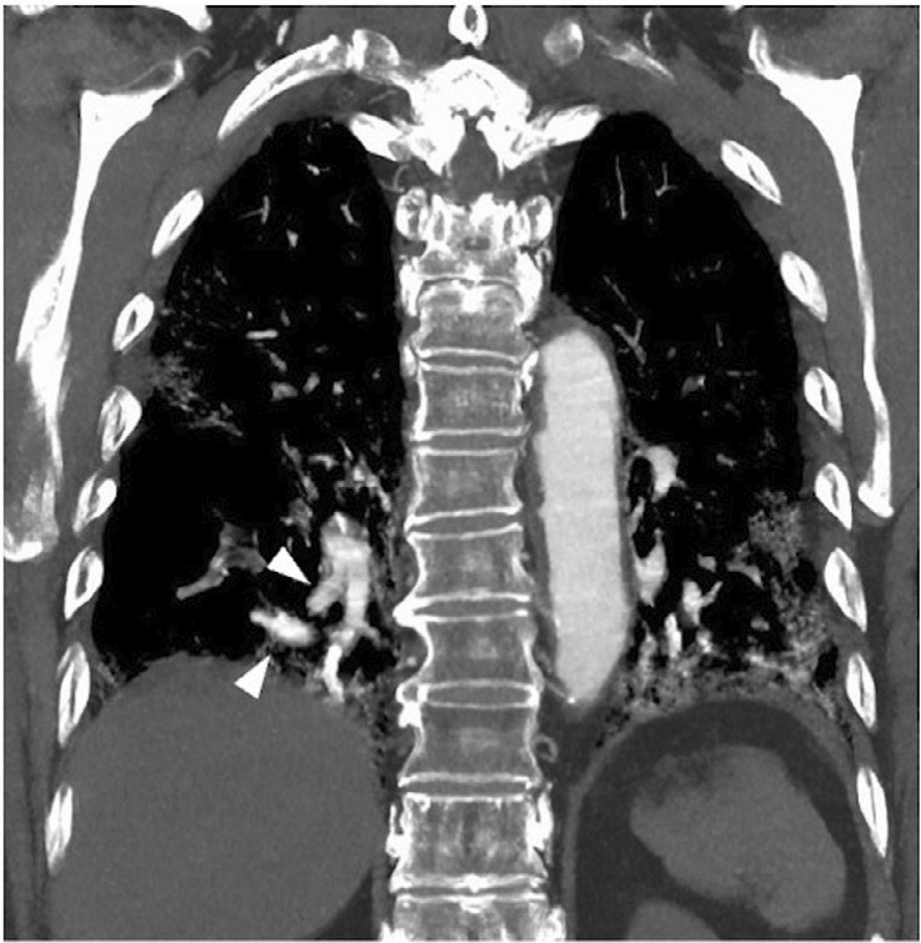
A contrast CT scan revealed an obvious vascular enlargement toward the lesions in the lower lobe bilaterally (arrowhead). CT, computed tomography
